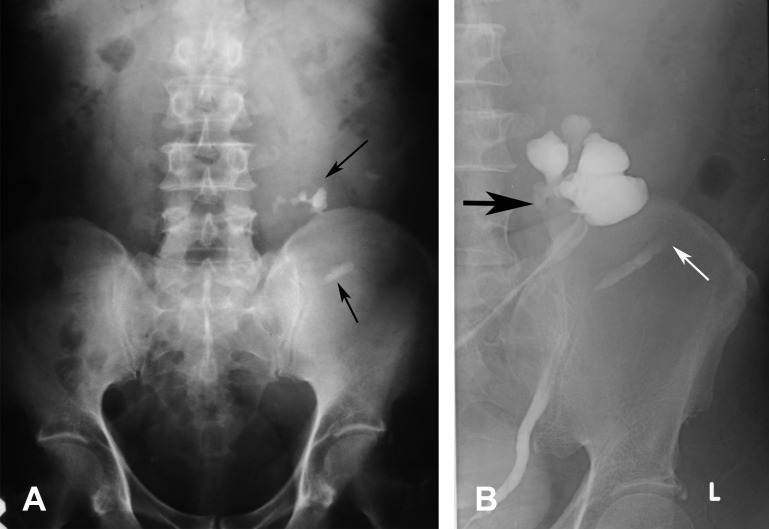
A 56-year-old man presented with fever and blurred vision. He was found to have diabetes mellitus, hypertension, and high creatinine level. (A) Abdominal radiograph shows multiple stones (arrows) in the left side of the abdomen which are lower than the normal renal position and nonvisualised right renal outline in the right renal fossa. US abdomen (not shown) showed no kidney in the right side and left hydronephrosis. (B) RP shows right crossed kidney with nonrotation and hydronephrosis (black arrow), and moderate hydronephrosis. The left ureter is laterally deviated with nonvisualised left kidney due to obstructed stone which is seen as a filling defect (white arrow).
